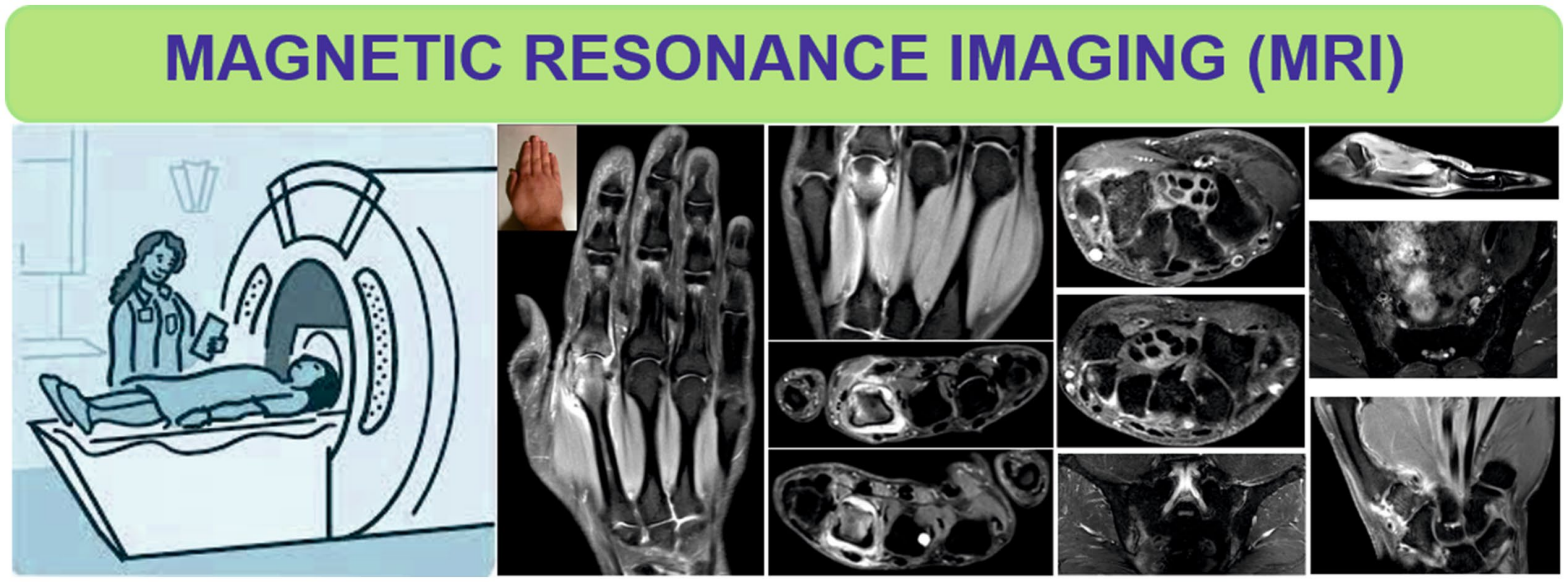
Magnetic resonance imaging of the hands and sacroiliac joints in patients with rheumatoid arthritis and axial spondyloarthritis.
